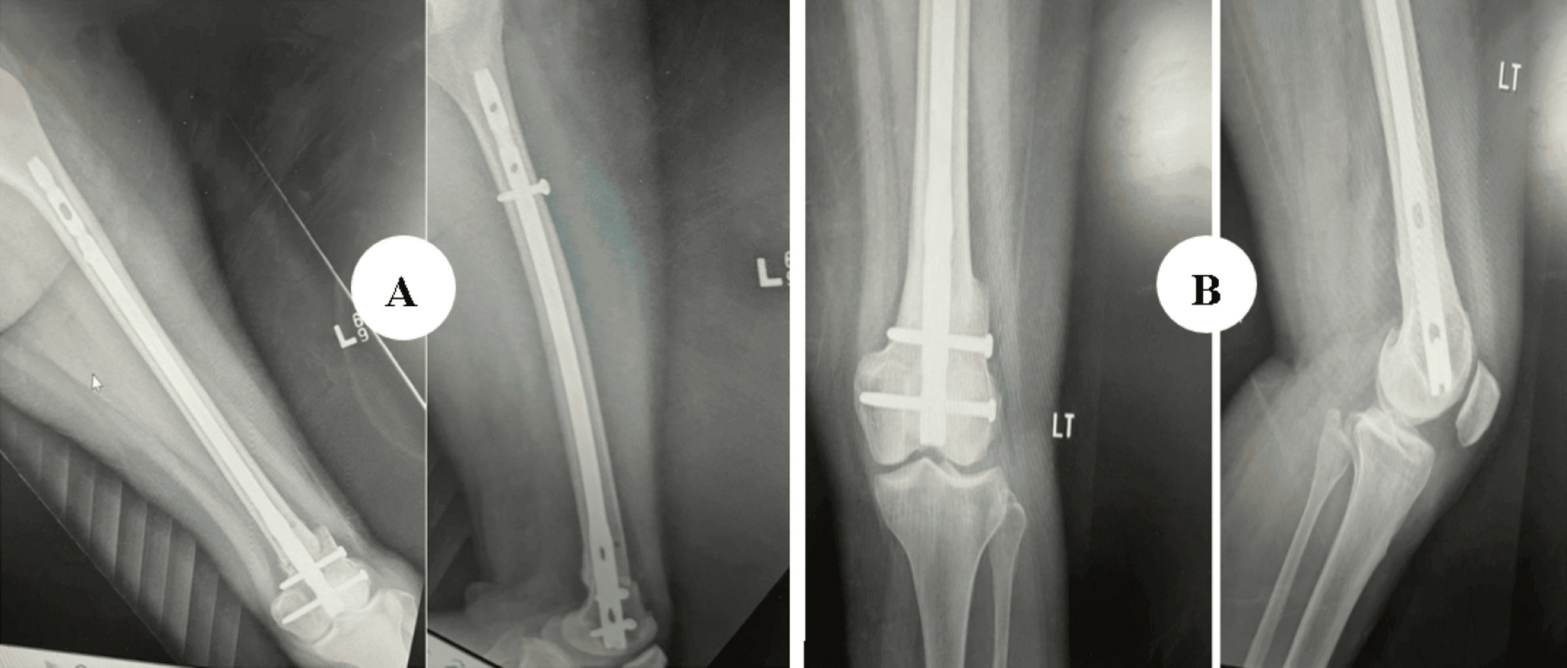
Case 4 follow-up X-rays at (A) three and (B) six months
